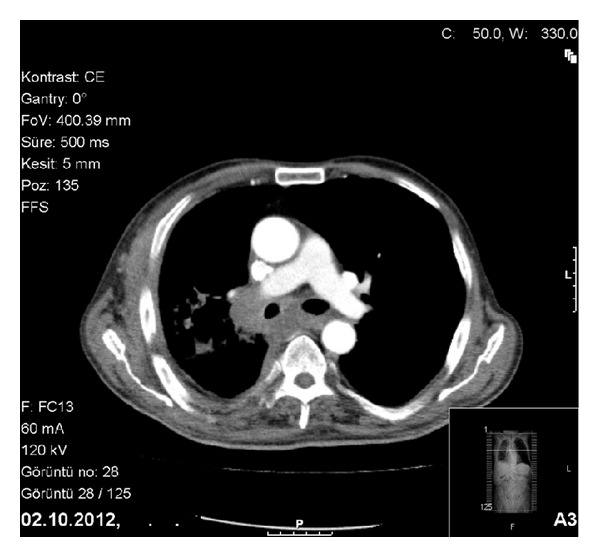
Subcarinal and right hilar lymphadenopathies in thorax CT.
